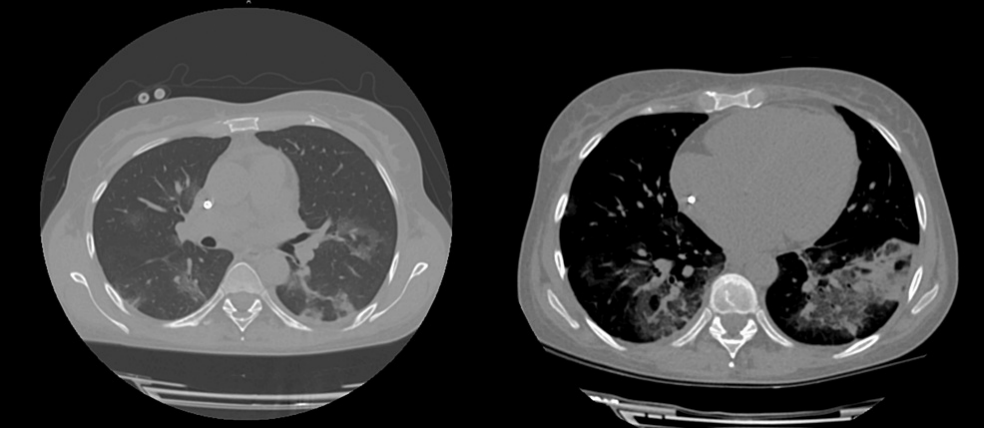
Chest CT taken on the sixth day of hospitalization
